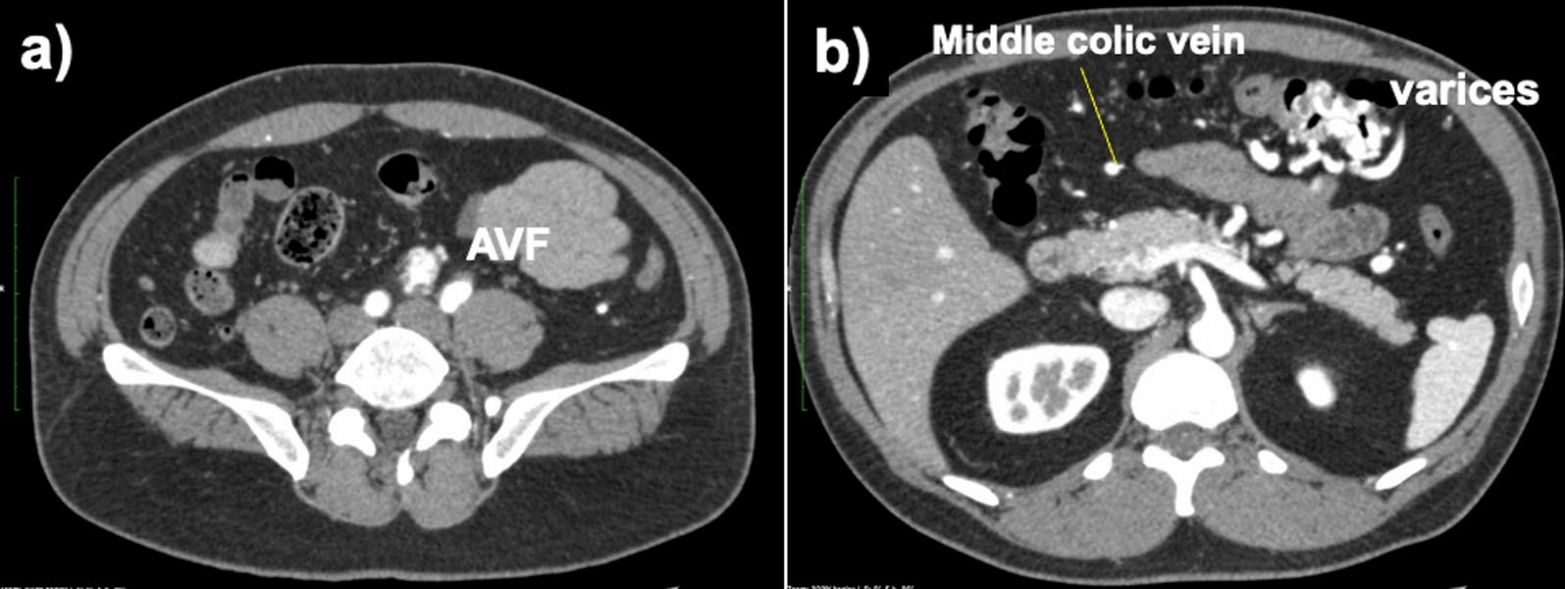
Contrast-enhanced CT was performed. a Early enhancement of the inferior mesenteric vein was observed as well as inferior mesenteric artery in the arterial phase. b Varicose veins were observed in the splenic flexure, communicating with the inferior mesenteric vein and the middle colic vein
